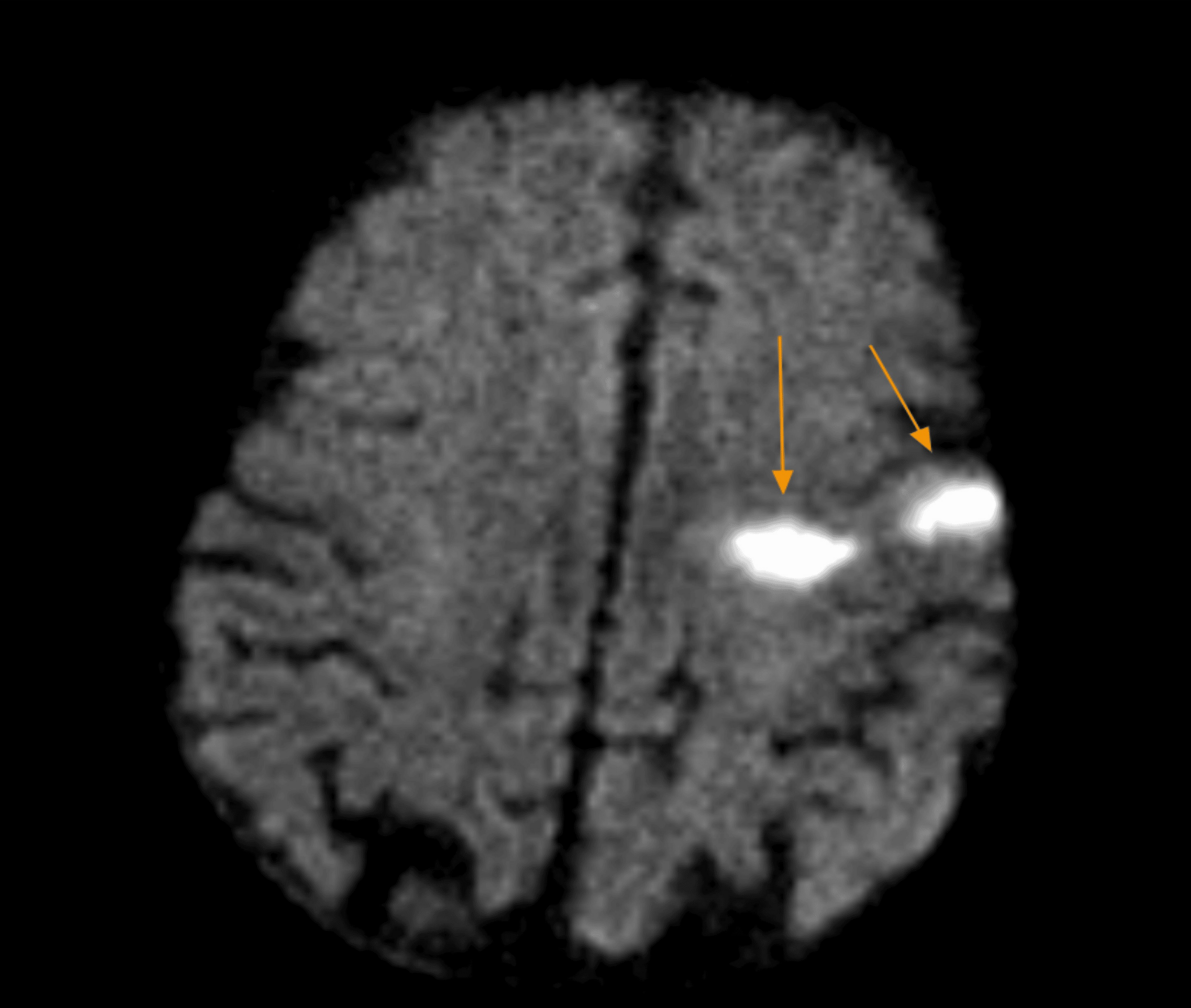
Diffusion-weighted imaging of the MRI brain showing a hyperintense lesion consistent with an infarct in the left precentral gyrus, as indicated by the orange arrows. MRI, magnetic resonance imaging
